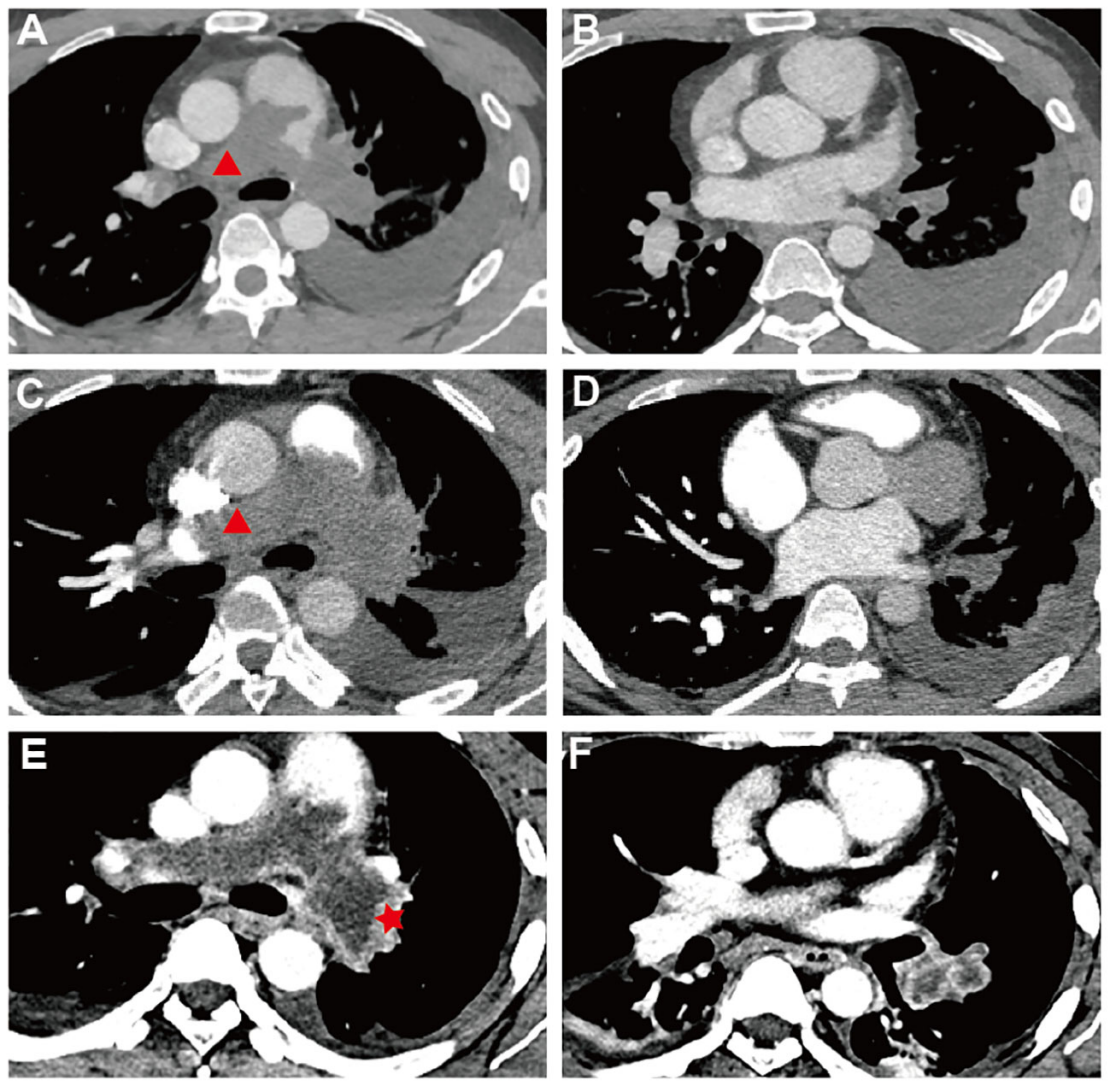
Pulmonary Computed Tomography Angiography| (A–F) Sequential PCTA Scans Demonstrate PAS Progression: (A, B) October 2022 - Low-density defects fill and expand both pulmonary trunks, eclipsing artery walls (red triangle) and causing left-sided pleural effusion; trunk diameter reaches 35 mm. (C, D) December 20–2 - Defects in pulmonary trunks enlarge, extending into the right ventricular outflow tract with progression beyond the left artery. (E, F) February 20–3 - Delayed-phase imaging reveals unevenly enhanced, irregular PAS lesions (red start), (F) the lesion within the branches of the left lower lobe arteries is markedly enlarged with inhomogeneous enhancement. (A, C) The red triangle marks the special PAS characteristics: complete arterial lumen filling with an “eclipsing vessel sign” and vessel expansion.
